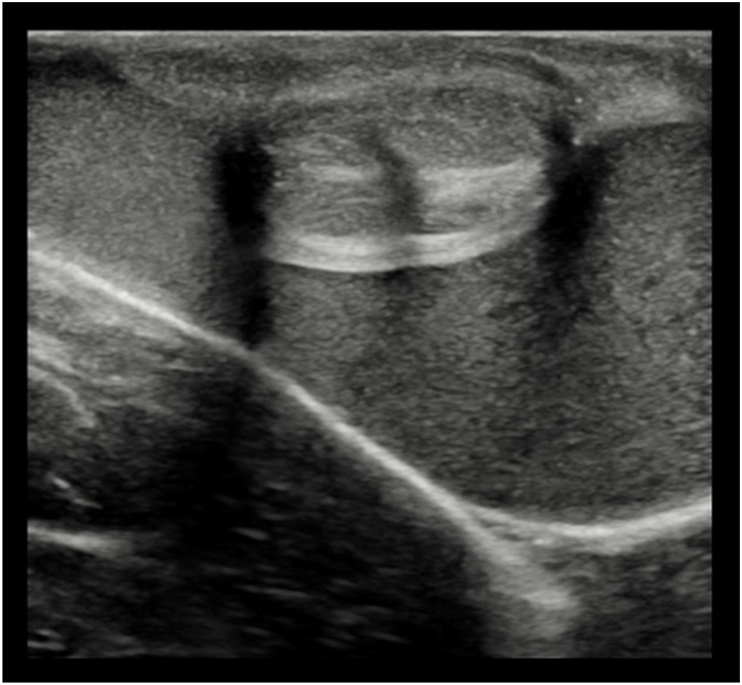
Longitudinal ultrasound of the penile shaft demonstrating a well-defined subcutaneous cystic lesion along the dorsolateral aspect (arrow). The lesion is hypoechoic with internal echogenic debris and posterior acoustic enhancement. No internal vascularity on color Doppler. The lesion is clearly separated from the corpora cavernosa and corpus spongiosum (asterisk), favoring epidermoid cyst.
